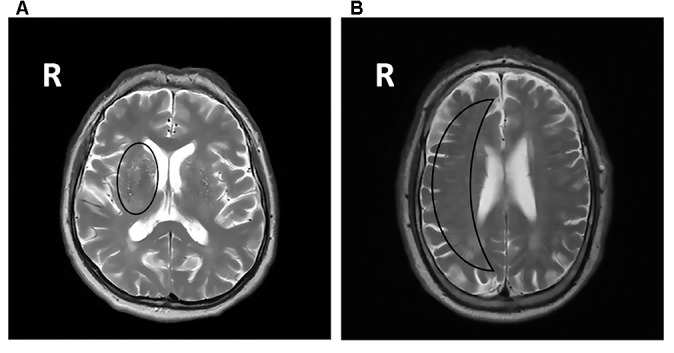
T2-weighted brain MRI scans showing symmetric extensive PVS at the level of the basal ganglia (A) and in the centrum semiovale (B). PVS, perivascular spaces; R, right.
